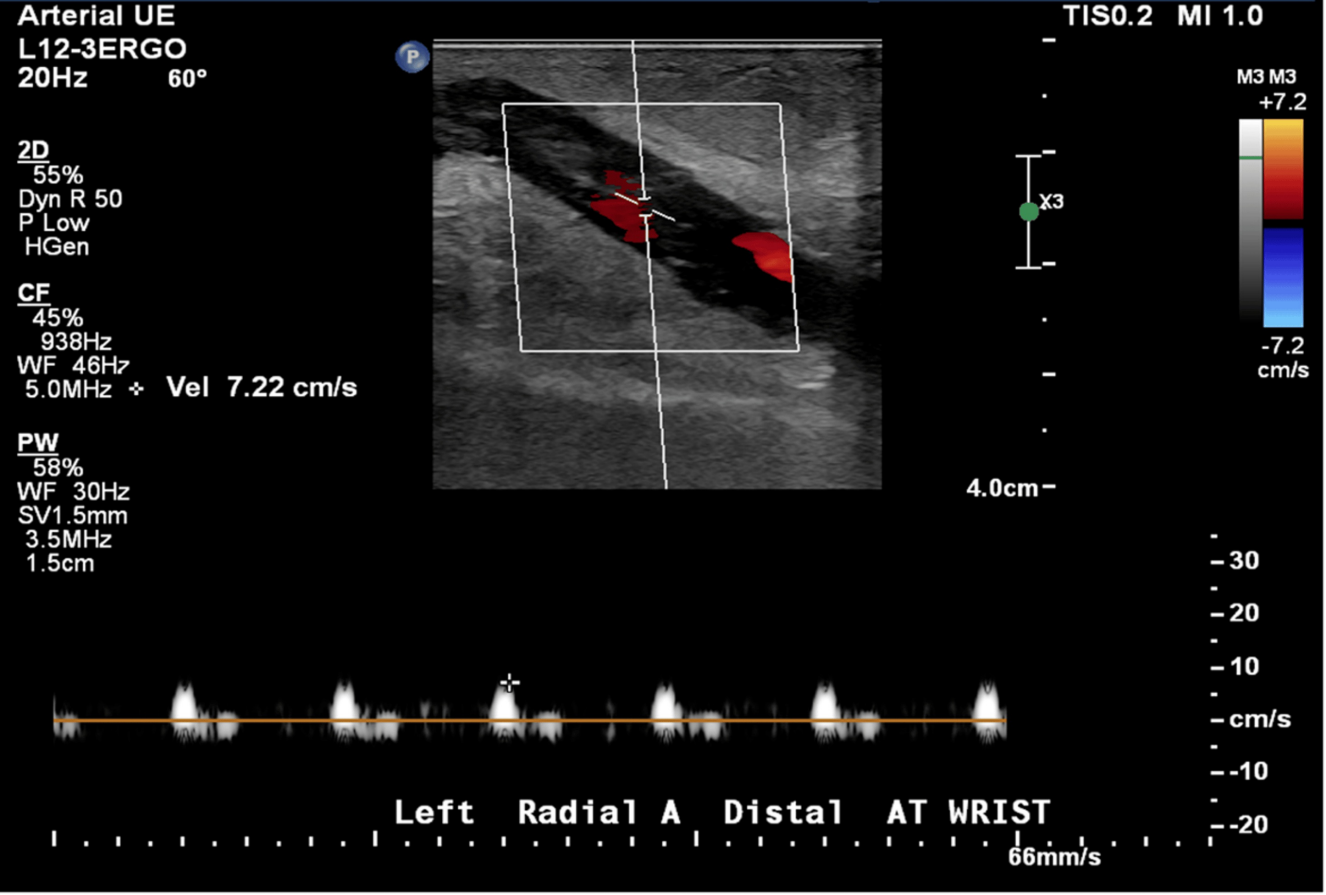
Left arterial upper extremity duplex ultrasound.
